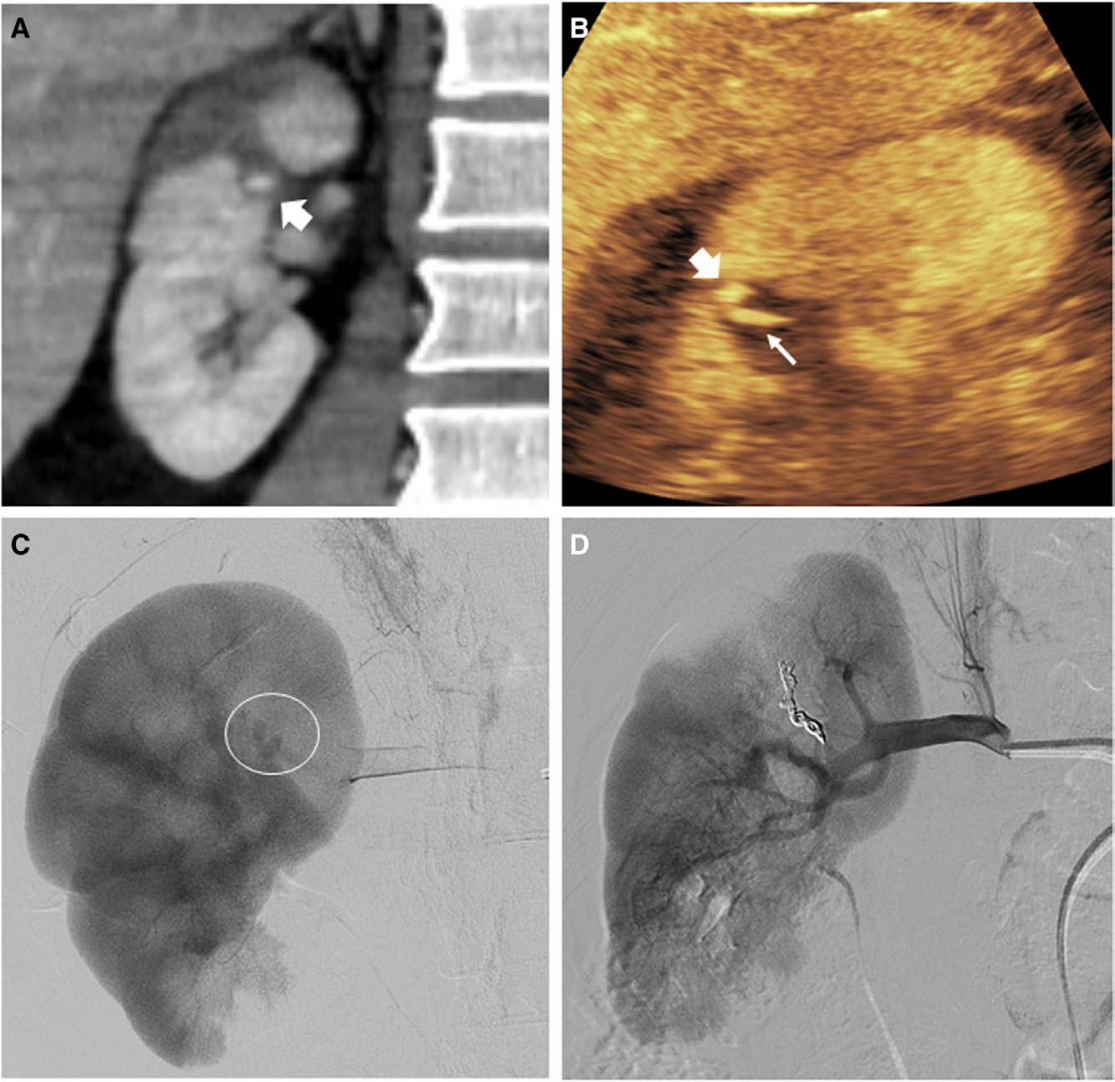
Segmental kidney infarction with combined vascular injury in a 46-year-old male after falling. (A) CT scan reveals segmental renal infarction with internal focal high-density foci suggesting pseudoaneurysm (arrow). An isolated pseudoaneurysm detected on CT may be observed for an increase in size as a conservative treatment. (B) Subsequent CEUS reveals a hyperechoic oval lesion with a short-tail sign suggesting pseudoaneurysm with low-velocity extravasation (thin arrow) requiring further evaluation, such as angioembolization. (C) The delayed image of the renal artery angiogram revealing a leaked contrast agent indicates that this is not an isolated pseudoaneurysm, which is consistent with the CEUS findings. (D) Complete total embolization of the renal artery was performed using coil and gel foam, and a subsequent angiography revealed successful complete obliteration of the renal artery. CEUS = contrast-enhanced ultrasound, CT = computed tomography.
